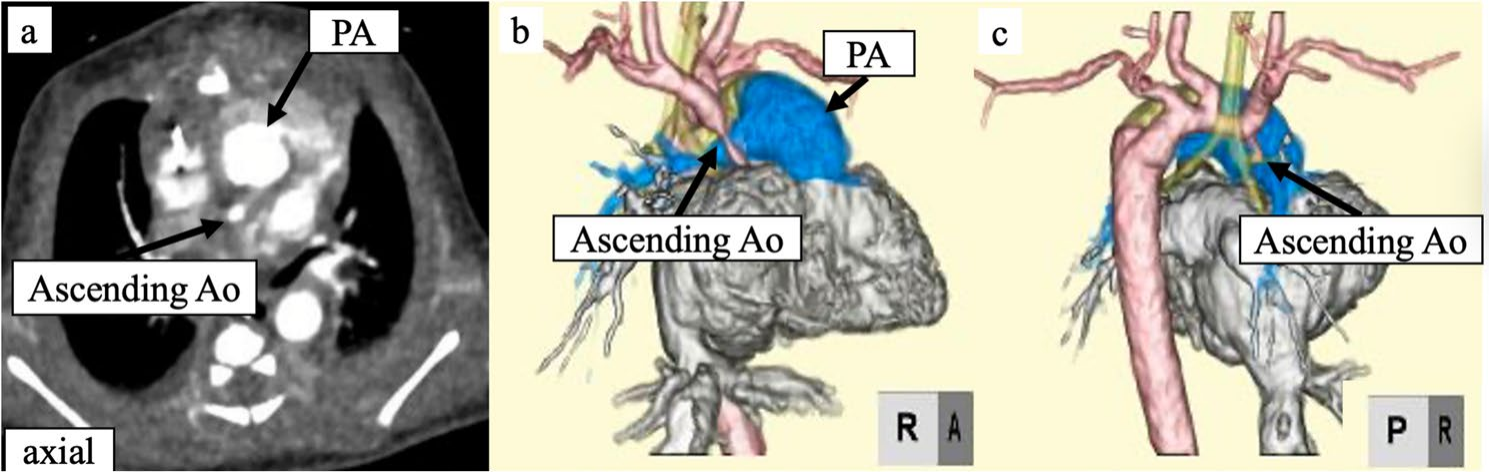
Preoperative computed tomography findings. a Contrast-enhanced axial scan. b and c Preoperative three-dimensional reconstruction images did not reveal a left coronary artery originating from the pulmonary artery. Ao, aorta; PA, pulmonary artery
